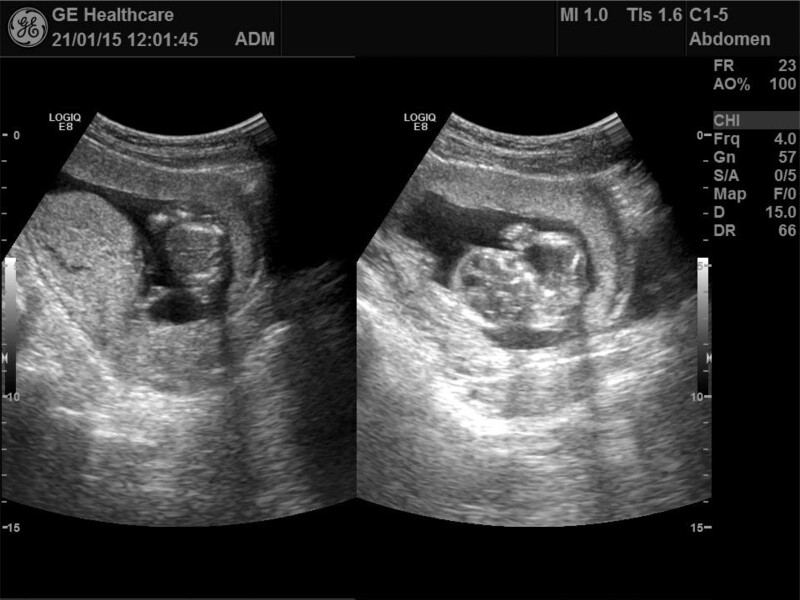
January 15, 2021: B-ultrasound displayed the resolution of the previously observed subclonial hematoma.
